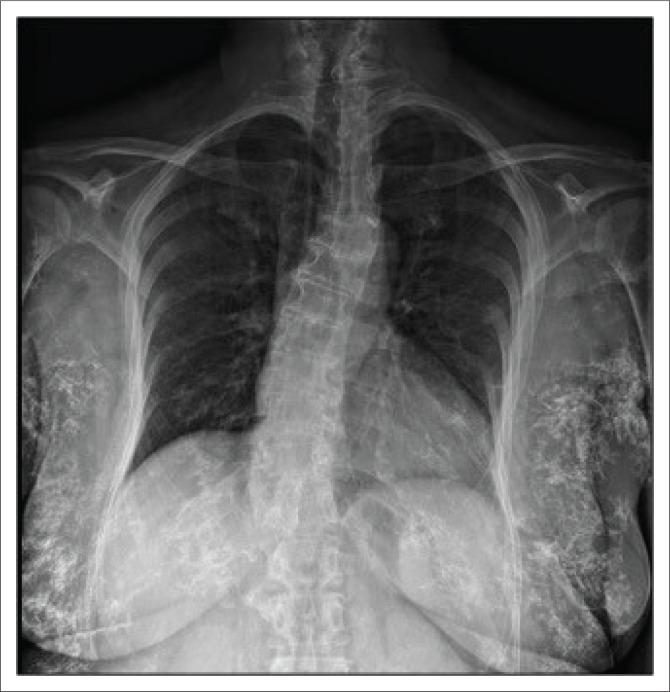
The chest radiograph (CHR) reveals the extensive bizarre breast calcifications with contrasting insignificant pulmonary changes.
